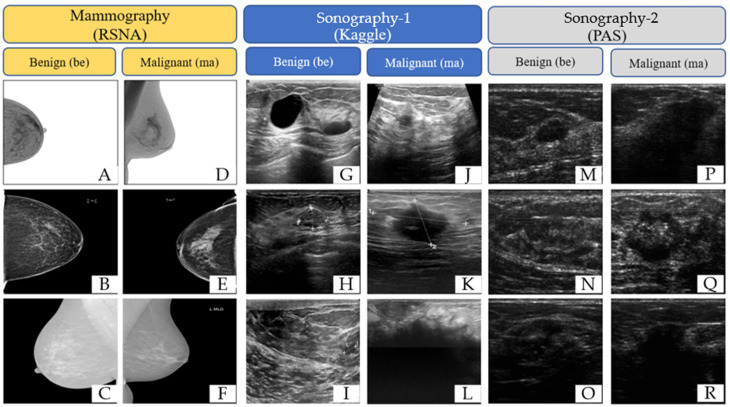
Representative examples of breast imaging modalities used in the study. The mammography (RSNA) for benign (A–C) and malignant (D–F) cases, sonography-1 (Kaggle) for benign (G–I) and malignant (J–L) cases, and sonography-2 (PAS) for benign (M–O) and malignant (P–R) cases.
